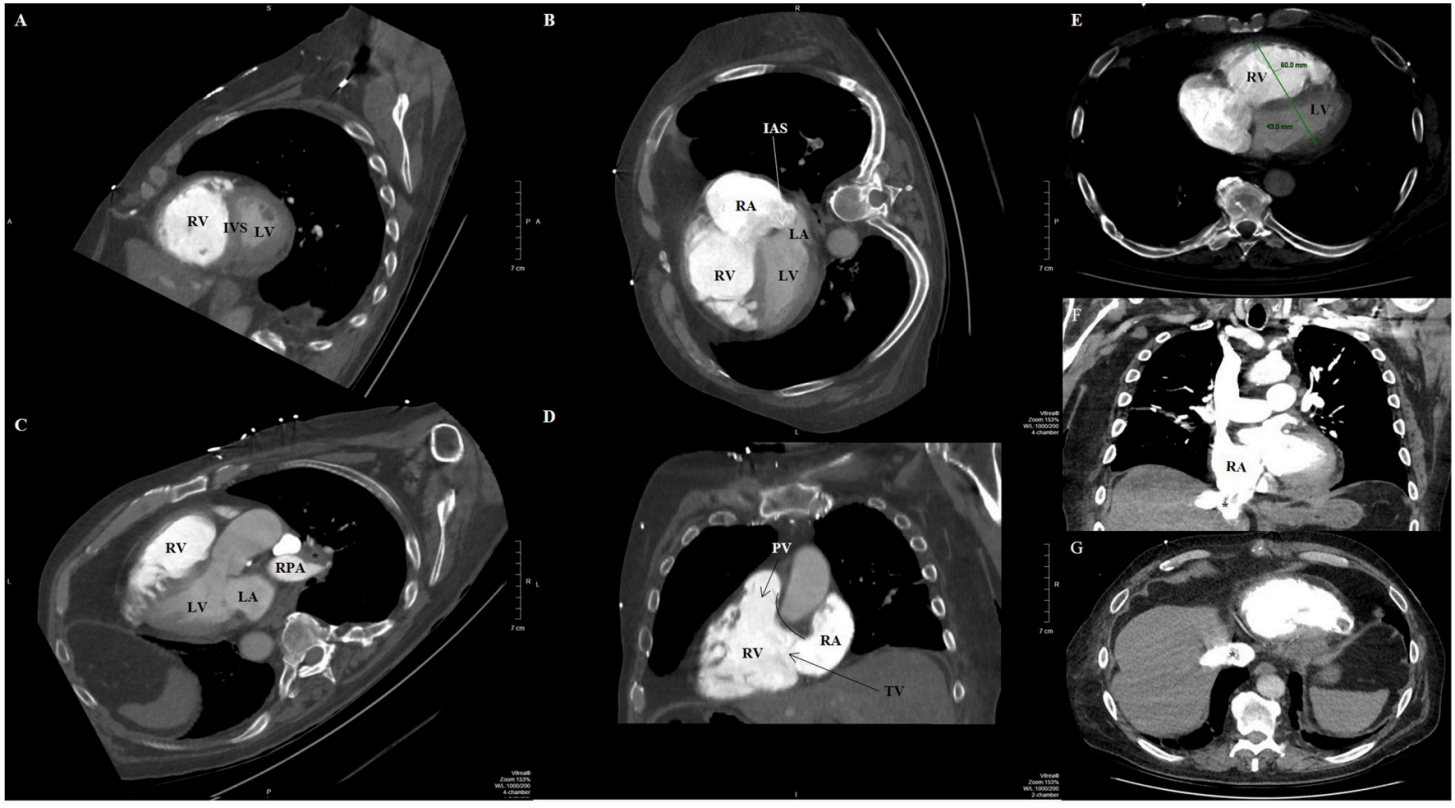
Contrast-enhanced computed tomography timed for the right heart and pulmonary artery circulation of a patient with pulmonary hypertension and pulmonary embolism, reformatted into cardiac views (A–D). (A) Short-axis view at the level of the ventricles reveals right ventricular dilation and interventricular septal flattening and bowing toward the left ventricle. (B) Four-chamber view showing both right ventricular and right atrial dilatation, and bowing of the interatrial septum. (C) Three-chamber view showing again enlarged right ventricle, as well as filling defect (asterisk) compatible with pulmonary embolism in the right pulmonary artery. (D) Two-chamber view dedicated to the right heart showing the right ventricular inflow and outflow, where the tricuspid and pulmonic valves can be seen, including the discontinuity between the atrioventricular (tricuspid) and semilunar (pulmonic) valves that characterizes the morphologic right ventricle. (E) Transaxial view showing increased RV:LV diameter ratio (1.4). Coronal (F) and transaxial (G) views showing contrast reflux (asterisks) into the inferior vena cava and hepatic venous system compatible with significant tricuspid regurgitation. IAS, interatrial septum; IVS, interventricular septum; LA, left atrium; LV, left ventricle; PV, pulmonic valve; RA, right atrium; RPA, right pulmonary artery; RV, right ventricle; TV, tricuspid valve.
